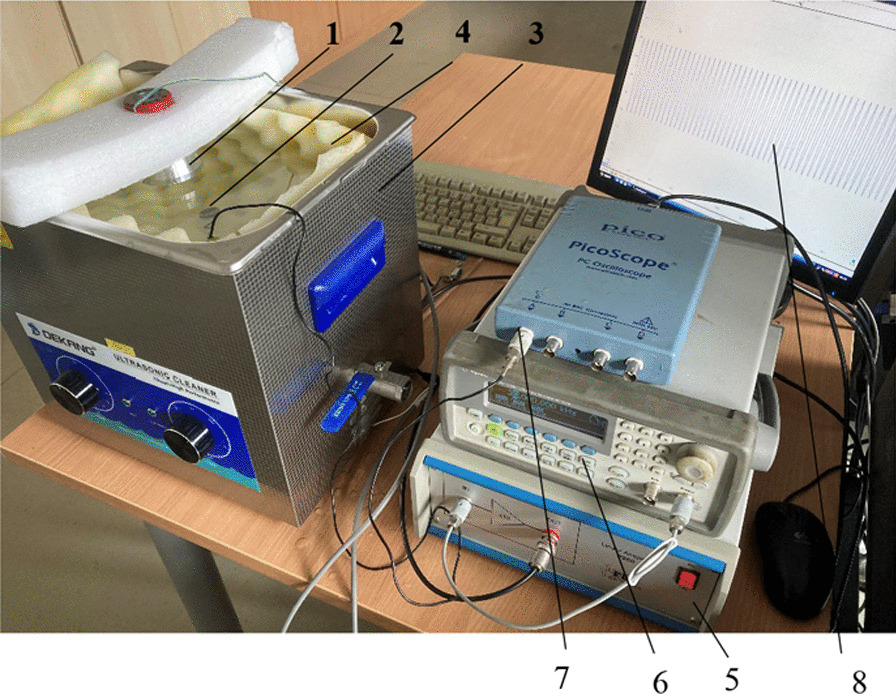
A set up for measurement of the acoustic wave pressure generated by the ultrasonic transducer: 1—ultrasonic transducer; 2—hydrophone; 3—water bath; 4—ultrasound attenuating material; 5—power amplifier P200 (FLC Electronics AB, Sweden); 6—signal generator Agilent 33220A; 7—oscilloscope PicoScope 3424 (PicoTechnology Ltd., UK) 8—PC
